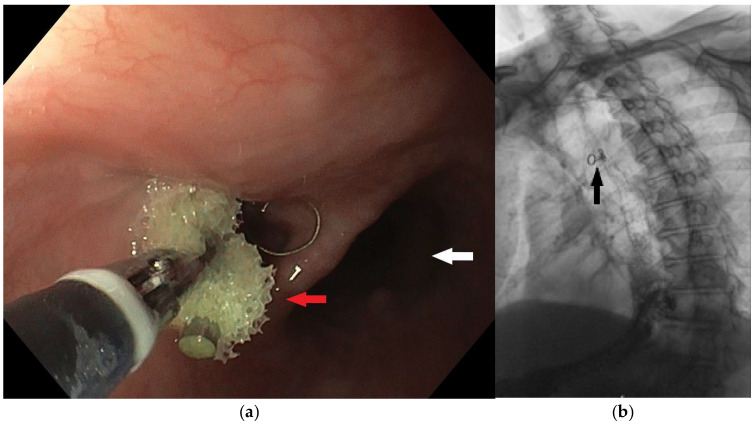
A metal coil (IMPEDE Embolization plug) was placed into the fistula tract (red arrow) by an interventional radiologist. The white arrow shows the lumen of the esophagus (a). The correct localization of the placed coil was confirmed by a chest x-ray (black arrow) (b).
